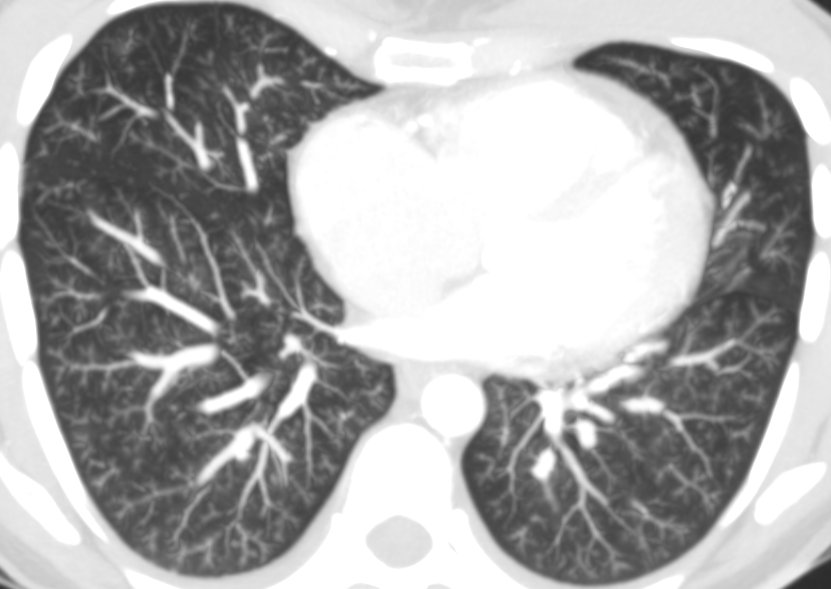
CT axial series of the lower lobes with maximum intensity projection showing diffuse centrilobular nodular opacities with ground-glass attenuation associated with tree-in-bud pattern.
